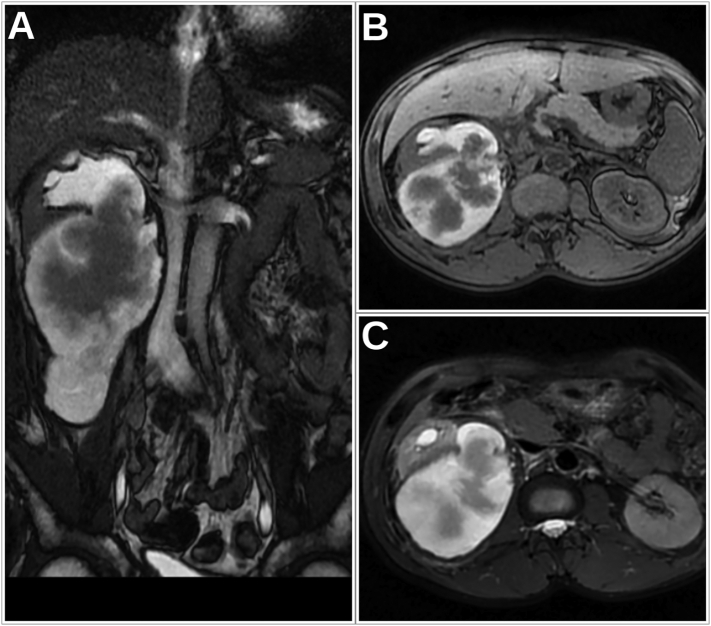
Abdominal MRI images: (A) A coronal FIESTA sequence showing the retrorenal collection emerging at the posterior wall of the pelvis; (B,C) An axial T1W and T2W with FatSat showing the hemorrhagic signal (high signal in both T1W and T2W FatSat sequences) in both the collection and the renal pelvis.
